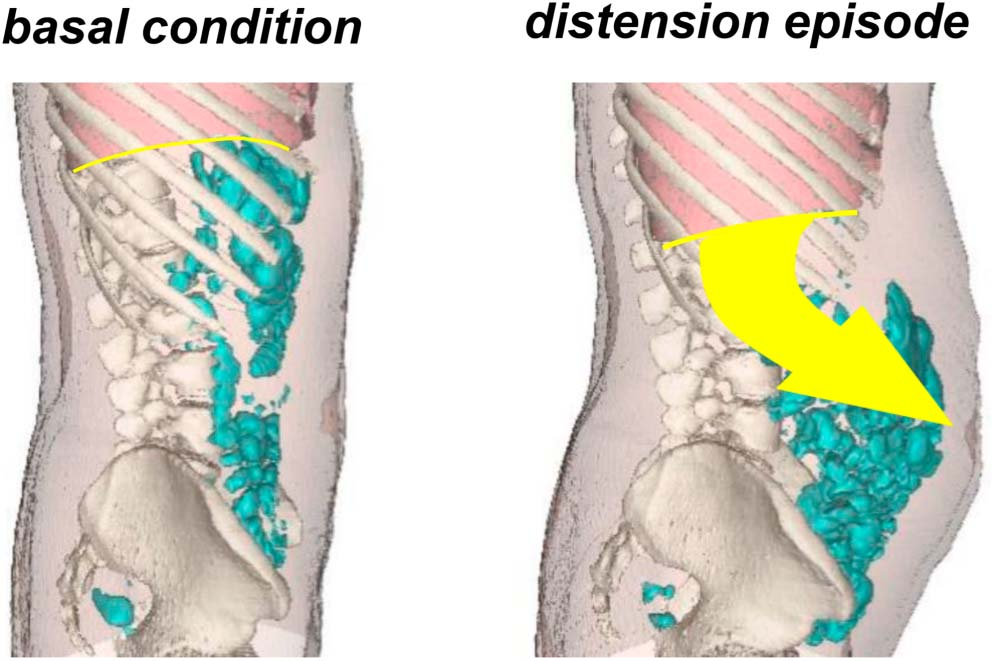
Example of abdominal distension. Three-dimensional reconstructions of CT scans obtained in the same patient during basal conditions and during an episode of abdominal distension. Note diaphragmatic descent and abnormal redistribution of abdominal content associated with distension. CT, computed tomography.
